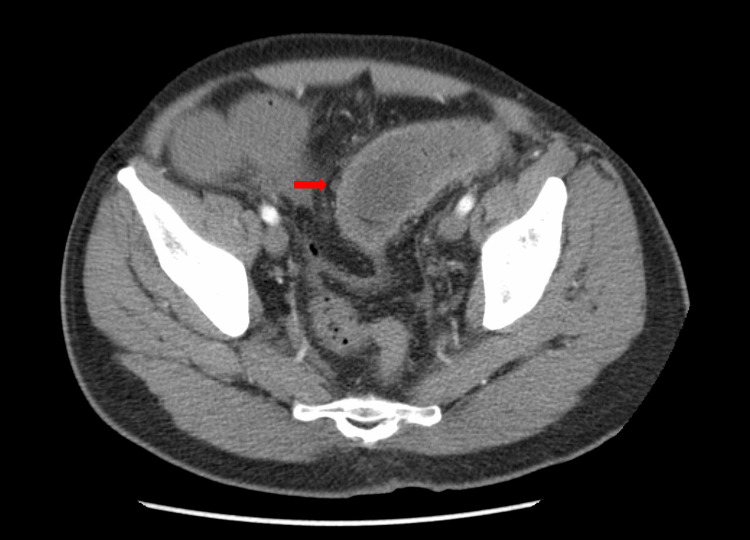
Transverse CT scan of the abdomen showing the gallstone ileus location. Reactive mural thickening and hyperemia extending to the splenic flexure were seen to the level of the obstruction. The red arrow indicates gallstone ileus. CT, computed tomography
